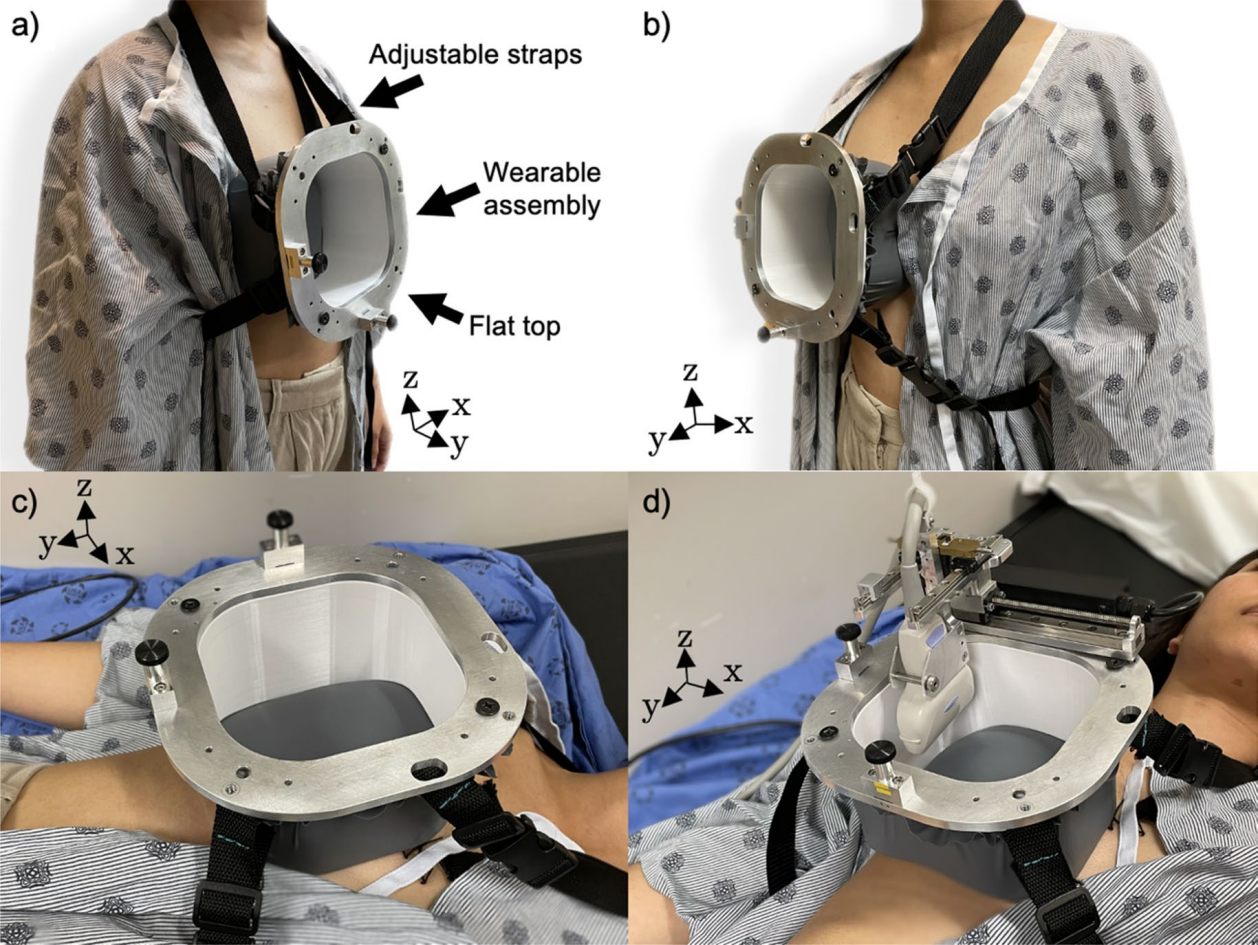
Images of the dedicated 3D ABUS system on a healthy female volunteer. Top Row: (a) 3D perspective view from a lateral camera position, showing the wearable 3D-printed dam assembly and disposable membrane attached onto the volunteer with the adjustable strapping mechanism; and (b) medial 3D perspective view from a medial camera position, showing that the contralateral breast is covered during acquisition. Bottom Row: Volunteer in supine position (a) without and (d) with the removable linear scanner for acquisition. Ultrasonic coupling gel would be placed inside of the dam and the ultrasound cradle would be adjusted prior to performing image acquisition.
